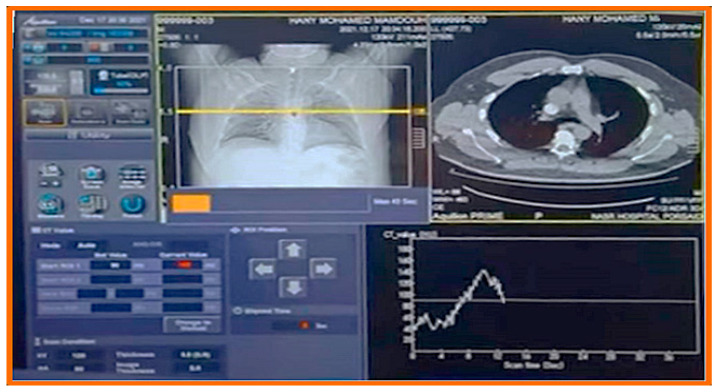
This image was taken of an obese patient who smokes and is over the age of forty. Axial CT pulmonary angiography (CTPA) slices show enhancement in the main pulmonary artery at 10, 30, and 60 s post-contrast injection. CT parameters: 120 kVp; 1.33 mm slice thickness; Bv40 reconstruction kernel; WL: 400, WW: 1000; scale bar: 5 cm. Representative non-study example (excluded case) shown for visualization of enhancement differences; this case was not included in the analyzed dataset according to exclusion criteria.
